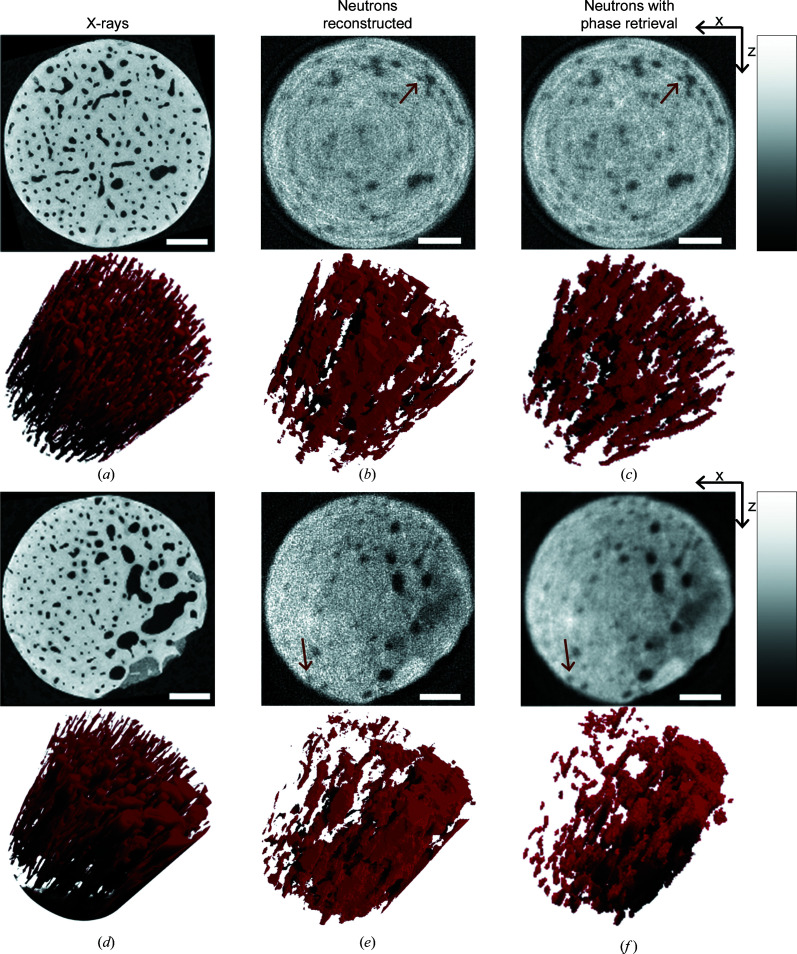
Bone samples. Typical cross sections and 3D visualizations of the void spaces for (*a*), (*d*) the laboratory-based microtomography X-ray data, (*b*), (*e*) the reconstructed neutron data and (*c*), (*f*) the phase-retrieved neutron data. The top panels show the bone sample composed of cortical bone only, while the bottom panels show the bone sample composed of more porous cortical bone. Scale bars represent 1 mm. Brown arrows point to finer features where phase retrieval enables a clearer identification from the noise. Corresponding 3D renderings of the segmented blood vessels are shown in red; images have been rotated to be displayed in the same orientation. The contrast in the images is enhanced by limiting the range of the red intensity scale from 2 to 98% of the maximum values in the data.
